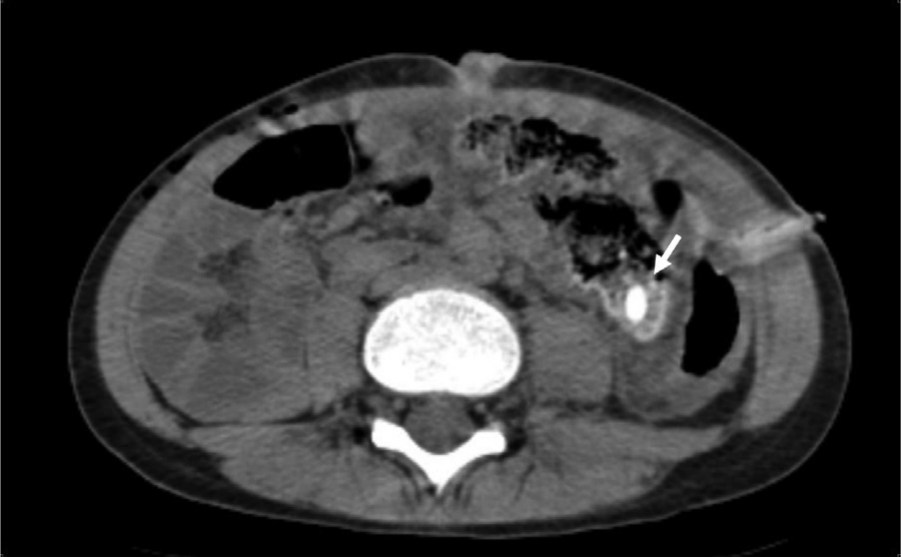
Postoperative abdominal CT scan showing migration of the stercolith in the cecum (white arrow).
